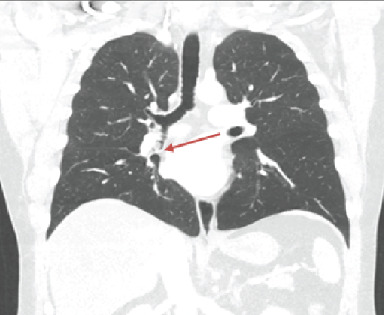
CT chest, coronal view: red arrow denoting another view of the exophytic soft tissue lesion.
